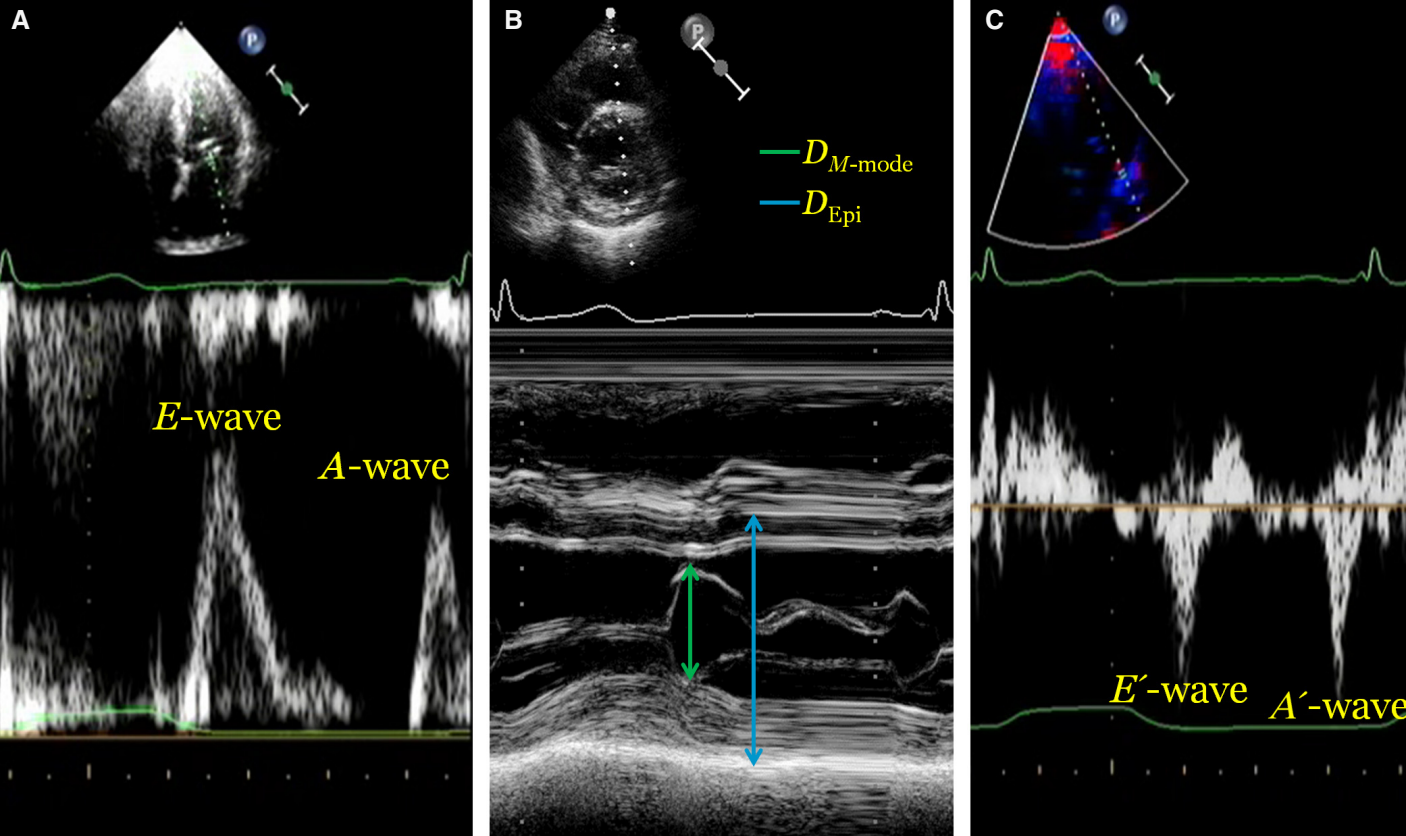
(A) Typical Doppler transmitral flow image showing one cardiac cycle with Doppler *E*- and *A*-wave marked. (B) Typical parasternal *M*-mode image with peak mitral leaflet separation (*D*_*M*__-mode_) in green and epicardial diameter (*D*_epi_) in blue. (C) Typical tissue Doppler, lateral mitral annulus motion image with Doppler *E′*- and *A′*-wave marked. See text for details.
